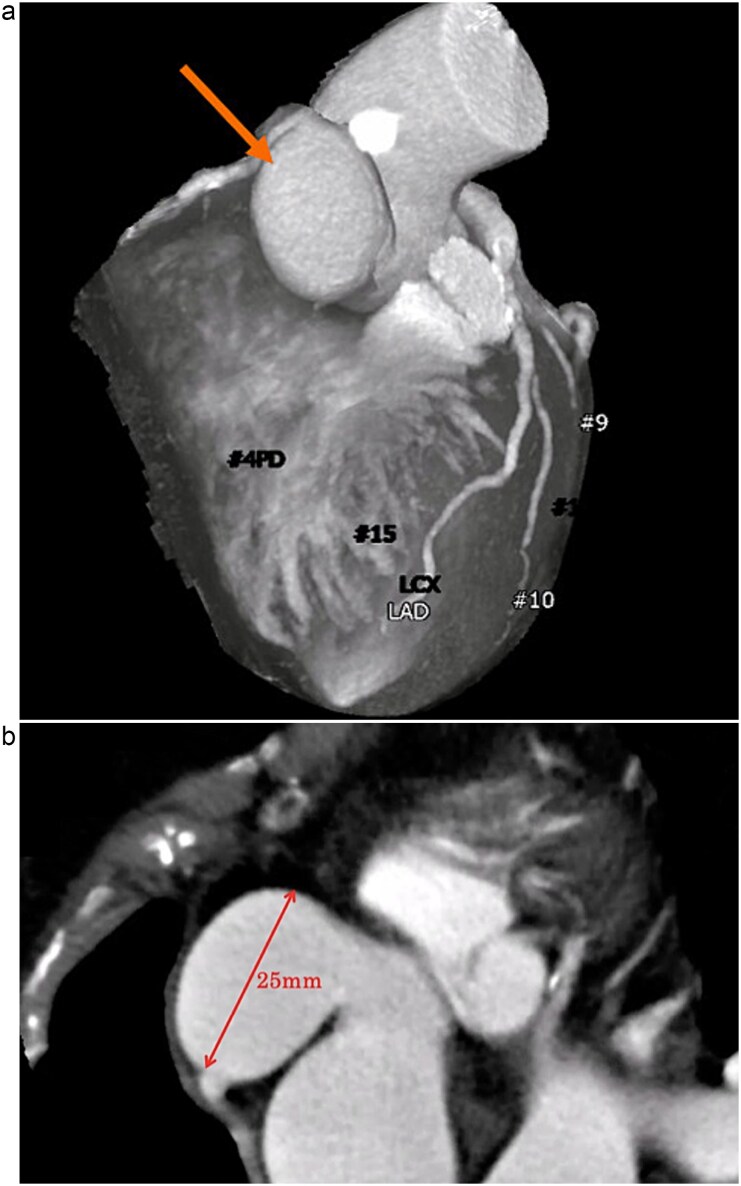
(a) Contrast-enhanced CT demonstrating a saccular aneurysm arising from the right coronary sinus of Valsalva (arrow), without evidence of significant coronary artery disease. (b) Axial contrast-enhanced CT image showing the saccular aneurysm, with a maximal diameter of 25 mm.
